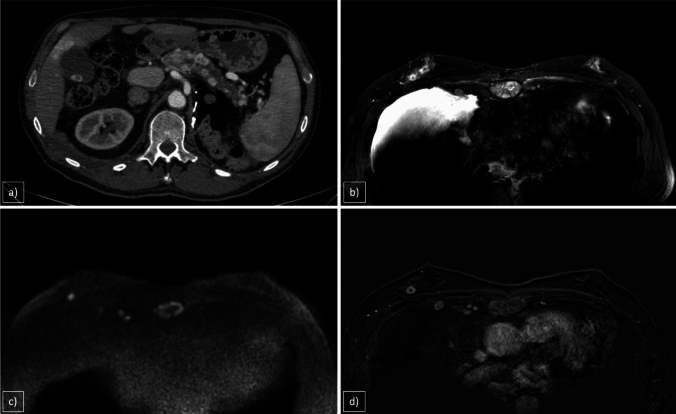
a Arterial Phase CT scan, b STIR, c DWI, d CE T1WI. 78-year-old man with a history of left nephrectomy for renal carcinoma and multiple pancreatic metastases. The patient complains about the appearance of a palpable solid nodule in the right breast. MRI reveals a solid lesion of 10 mm with central necrosis on STIR sequences, accompanied by pleural effusion and pleural nodules. These pleural nodules demonstrate restricted diffusion and post-contrast rim-enhancement, along with evidence of bone remodeling at the sternal level. An US-guided biopsy confirmed the diagnosis of renal carcinoma metastasis
